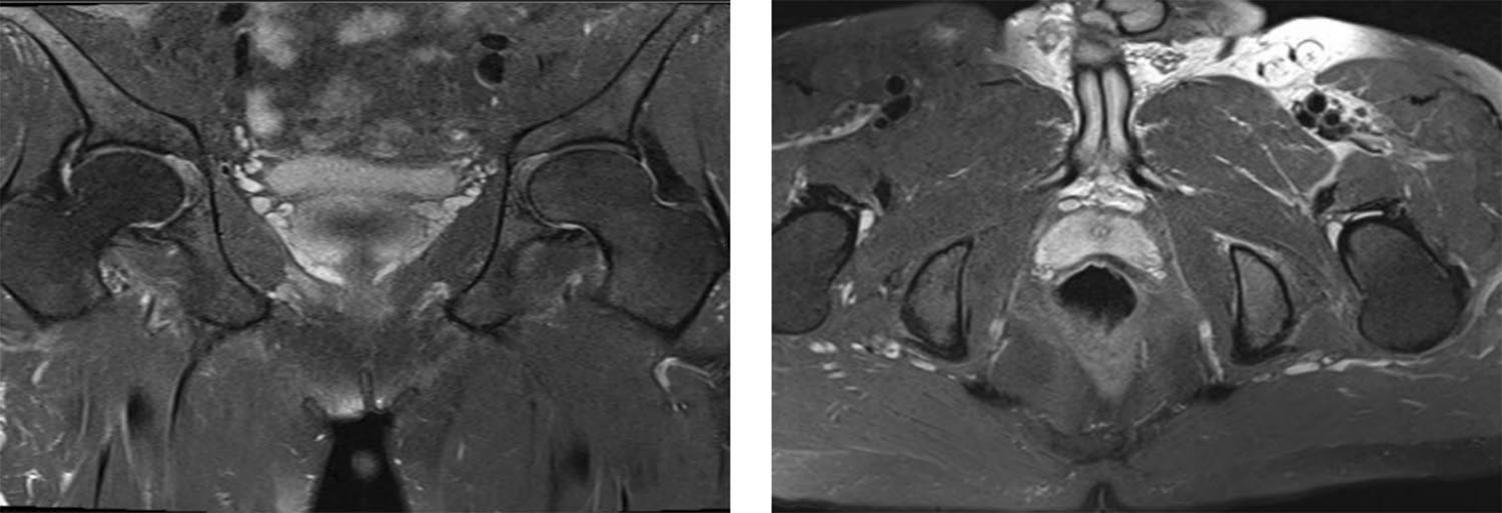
Perianal magnetic resonance imaging (MRI) 4 months after the operation. The long fistula tract was disappeared. The perianal MRI shows excellent healing
